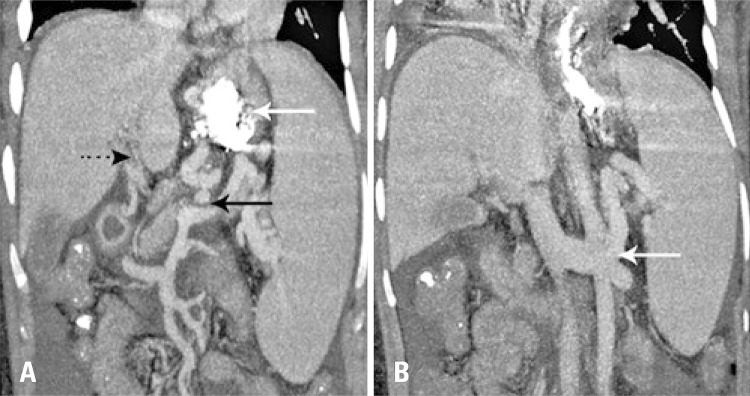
Computed tomography with intravenous contrast. (A) Enlarged left gastric vein (continous black arrow), portal thrombosis with cavernomatous transformation (pointed black arrow) and signs of previous gastresophasic varices embolization (white arrow). (B) Splenorenal shunt (white arrow)
